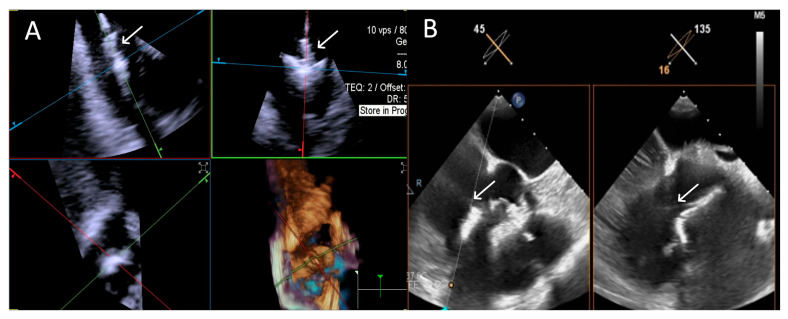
Step 4: insights from Case 3. The figure shows a comparative analysis of the trajectory evaluation of the delivery catheter (white arrow) during Step 4. In this case, the 4D-ICE (A) system offers better image quality, as TEE (B) was affected by shadowing artifacts from anterior structures. Abbreviations: 4D-ICE: four-dimensional intracardiac echocardiography. TEE: transesophageal echocardiography.
